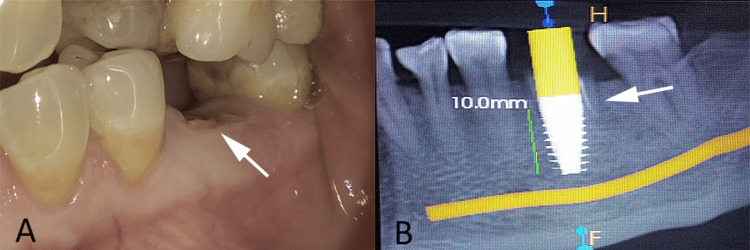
Clinical and radiographic evaluation findings A: clinical view showing a grossly decayed mandibular left first molar with residual root stumps (white arrow); B: preoperative radiograph demonstrating adequate interradicular and apical bone for immediate implant placement (white arrow). Original patient images, used with the patient's permission.
